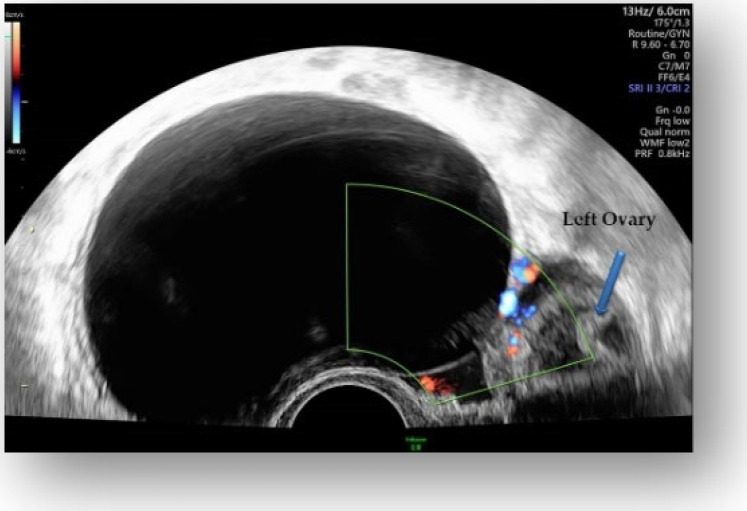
Hypoechoic cystic formation with peripheral doppler flow, without intracystic vegetations.
